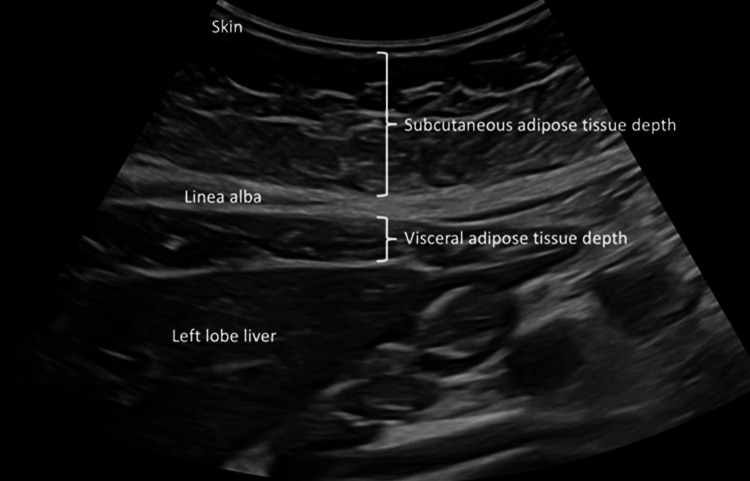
Ultrasonographic measurement of subcutaneous and visceral adipose tissue thickness Image credit: Sawanya Benchahong
